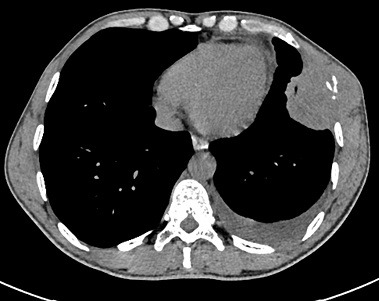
Chest CT scan showing a heterogeneous mass localized in the 6^th^ left rib
